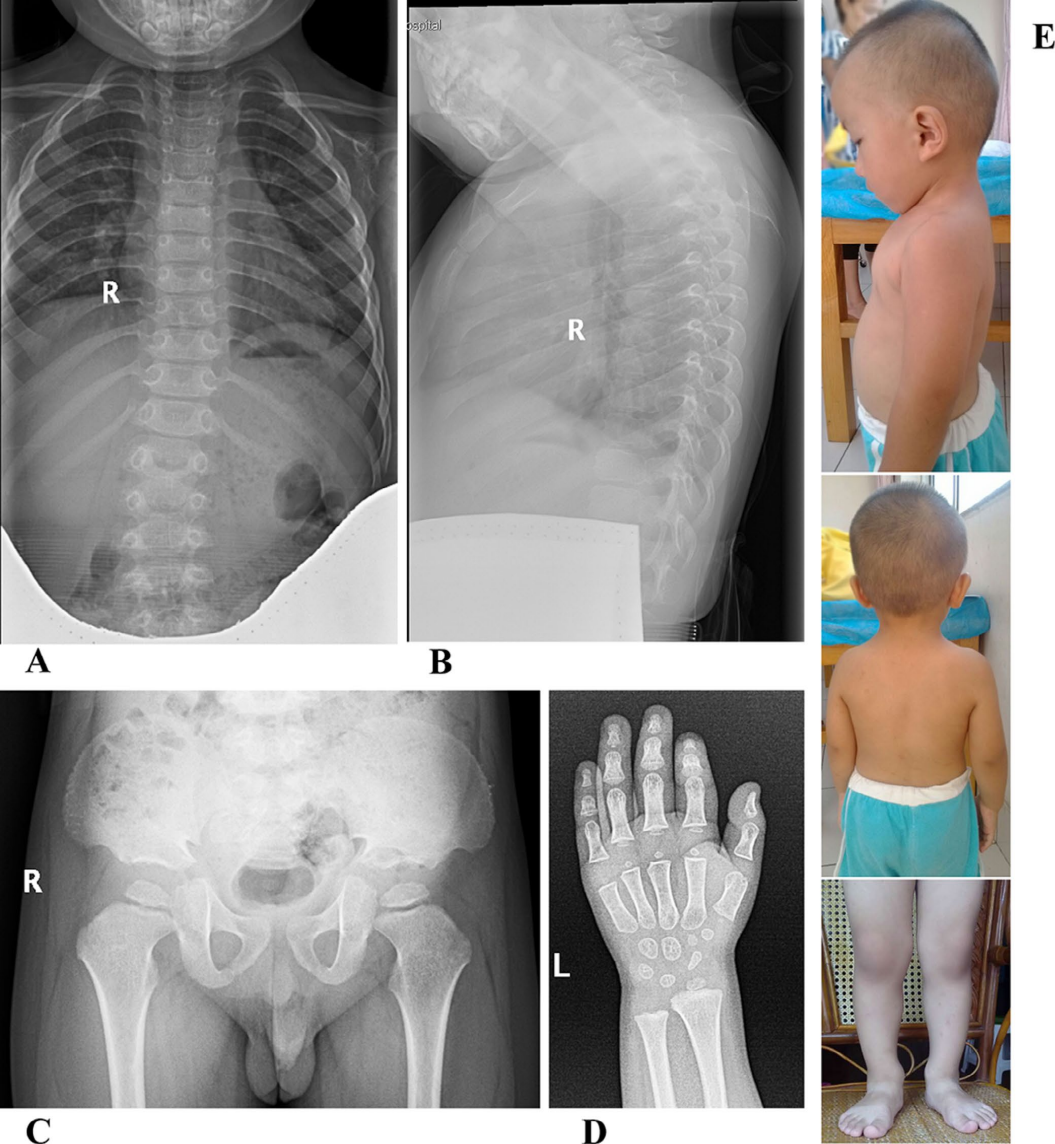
Clinical and radiographic manifestations of the child at the age of 2 years and 9 months. (A, B) Anterior and lateral views of the spine. (C) Anterior view of the hip. (D) Anterior view of the hand. (E) Clinical features of the child.
